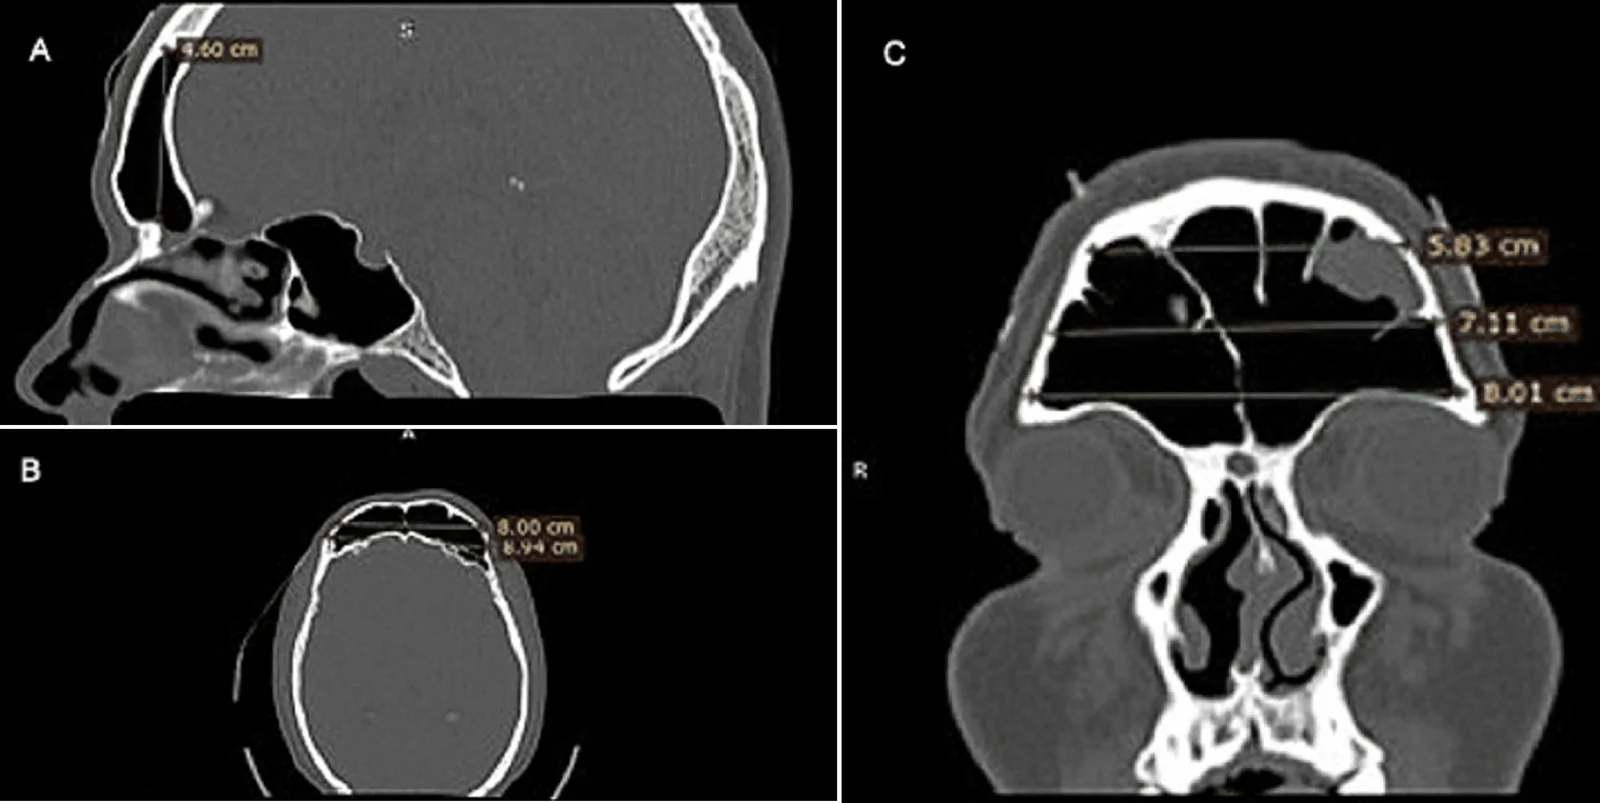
Non-contrast CT scan of nose and paranasal sinuses depicting pneumosinus dilatans (A) Sagittal view showing craniocaudal dimension of 4.60 cm; (B) Axial view showing average transverse dimension of 8.47 cm, and (C) Coronal view depicting average transverse dimension of 6.98 cm of the frontal sinus.
